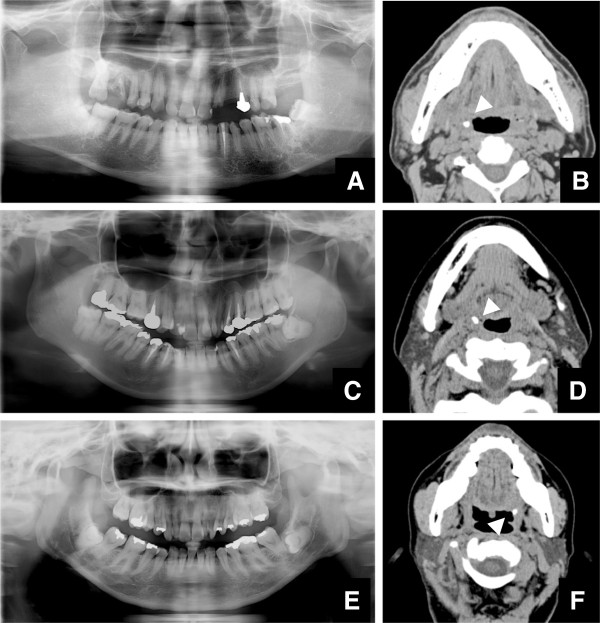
Cases with tonsilloliths are detected on CT images, but not on panoramic radiographs. A) Panoramic radiographs at the palatine tonsillary level of a 65-year-old male. A radiopaque nodular mass is not seen. B) Axial CT images at the palatine tonsillary level of the same patient as in Figure 5A. Tonsilloliths (arrowheads) are seen at the level of the mandibular teeth as high-density, oval-like structures with a long axis of 5.03 mm and 1128 HU. C) Panoramic radiographs at the palatine tonsillary level of a 32-year-old female. A radiopaque nodular mass is not seen. D) Axial CT images at the palatine tonsillary level of the same patient as in Figure 5C. Tonsilloliths (arrowheads) are seen at the level of the mandibular teeth as high-density, oval-like structures with a long axis of 5.96 mm and 912 HU. E) Panoramic radiographs at the palatine tonsillary level of a 48-year-old female. A radiopaque nodular mass is not seen. F) Axial CT images at the palatine tonsillary level of the same patient as in Figure 5E. Tonsilloliths (arrowheads) are seen at the level of the mandibular teeth as high-density, oval-like structures with a long axis of 5.35 mm and 905 HU.
